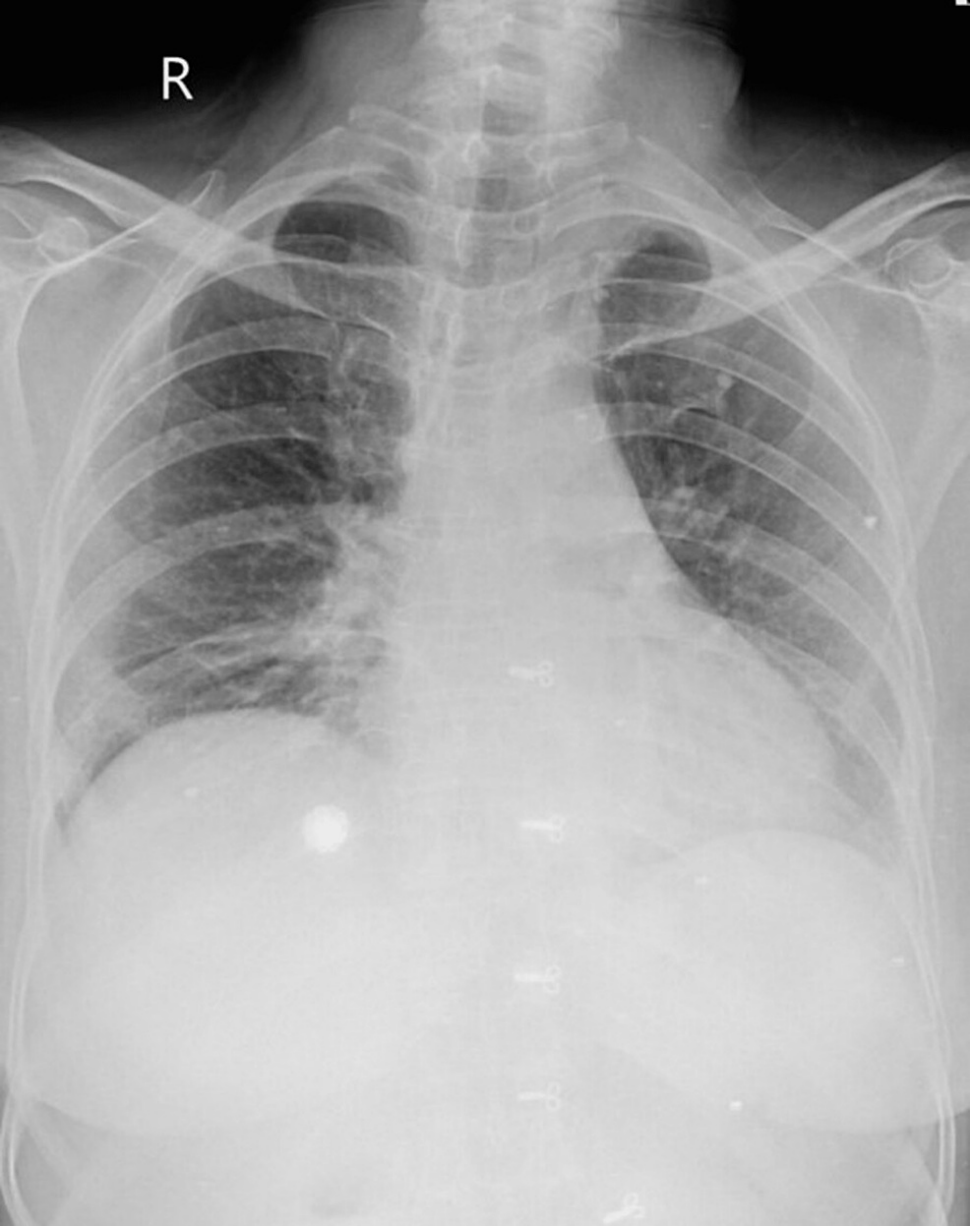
Chest X-ray of the patient on the day of discharge from the hospital.
